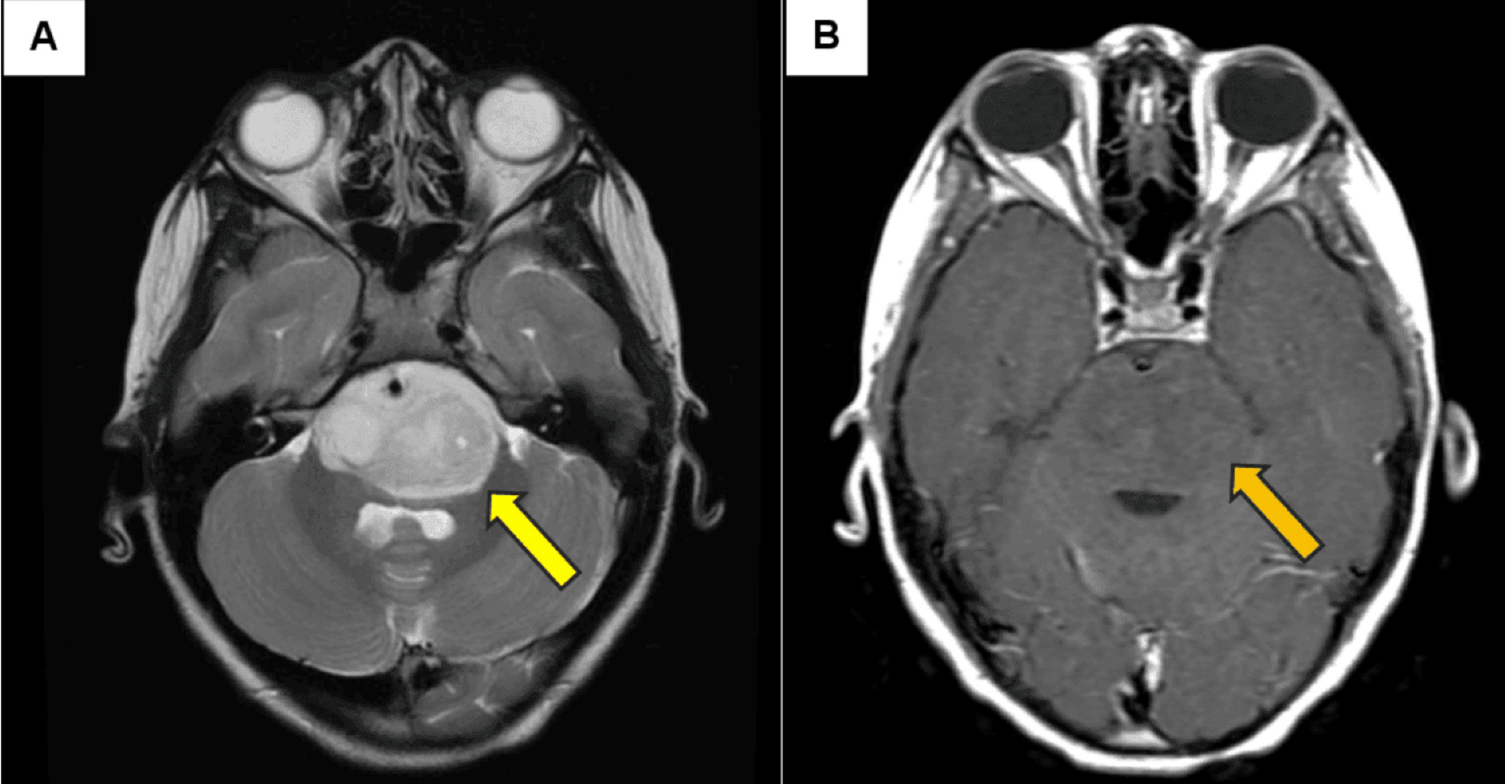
Axial T2 MRI showing a hyperintense brainstem lesion (A: yellow arrow) and T1 MRI displaying a subtle hypointense brainstem lesion (B: orange arrow). These MRI findings are consistent with a brainstem glioma.
